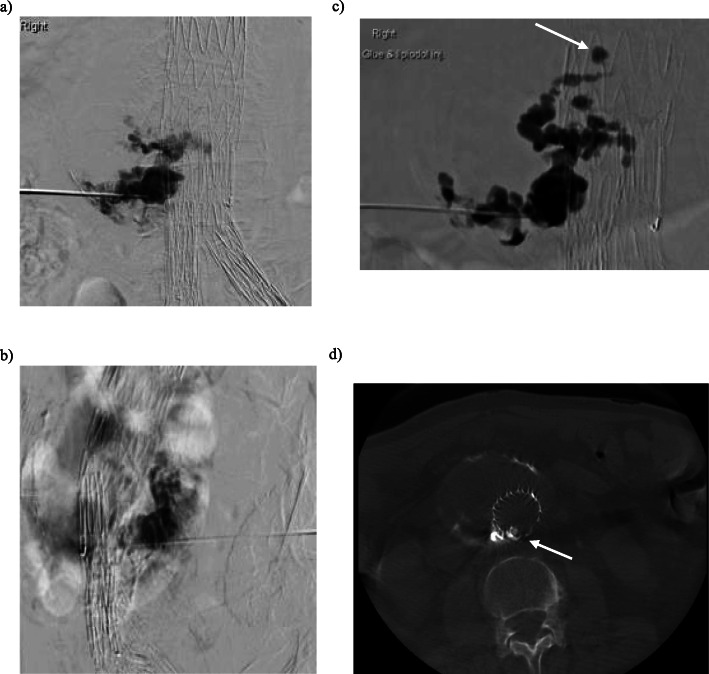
a/b Prone PA and lateral digital subtraction angiogram of the abdominal aortic sac injection with iodinated contrast through a 20G access needle demonstrates filling of the aortic sac and no feeding artery. c Prone roadmap image of the aortic sac demonstrates filling of the sac with 4:1 Lipiodol/Glue mix (arrow) and penetration through the fabric into the lumen of the graft (arrow). d Axial non-contrast cone beam CT (prone) of the abdominal aorta demonstrates non-target embolisation in the body of the stent graft. (arrow)
